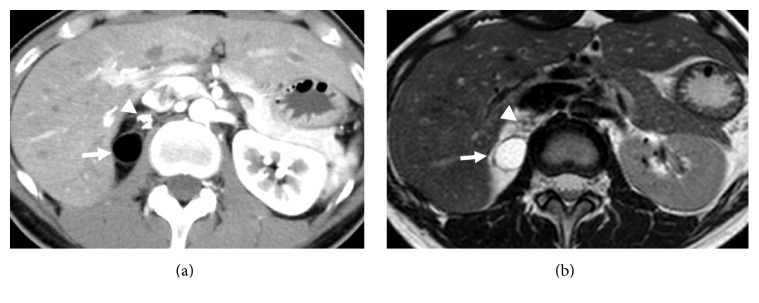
CT and MRI images of the tumor. Fat (white arrow) and calcification (white arrowhead) are shown in axial CT (a) and T2-weighted MRI (b) images.
